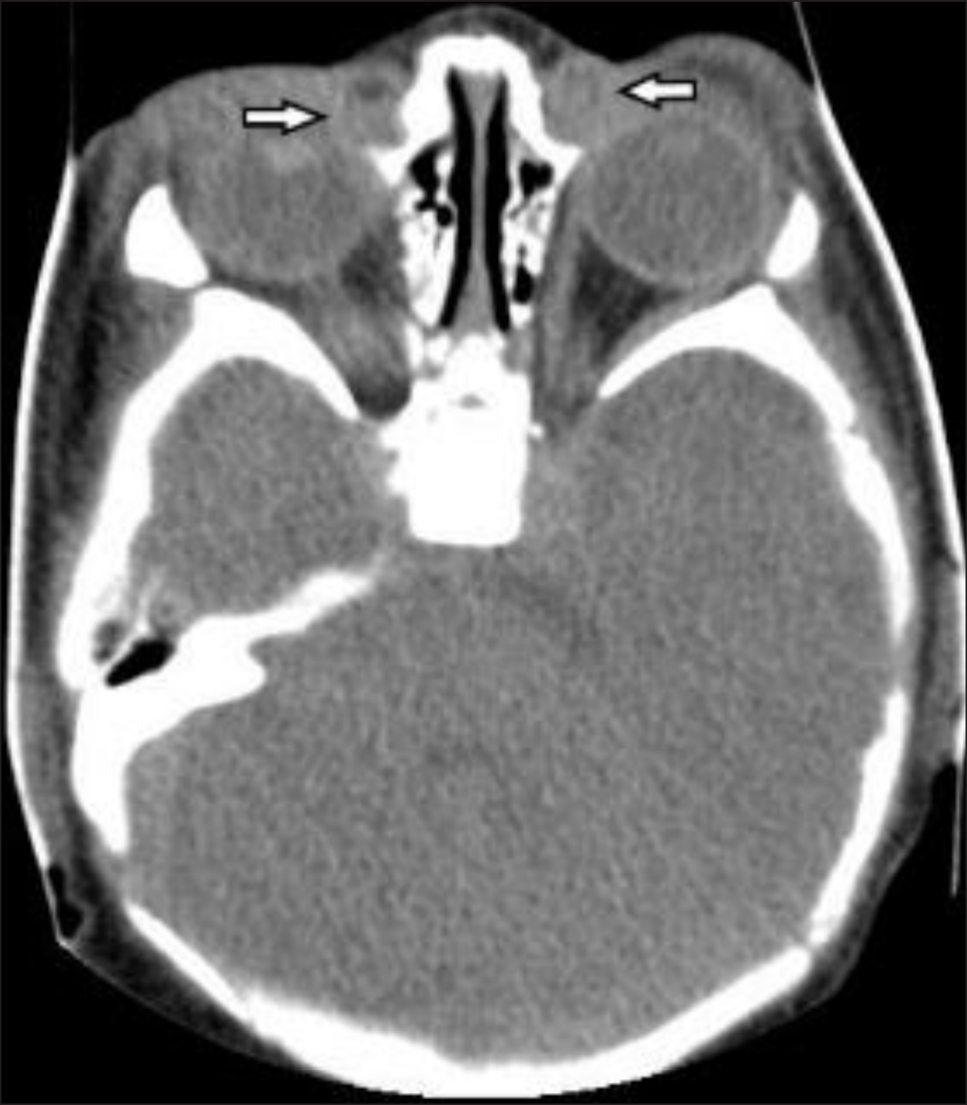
Axial CT image shows a bilateral soft tissue mass (arrows) at the level of the medial canthus, which is formed by the dilated lacrimal sac.
